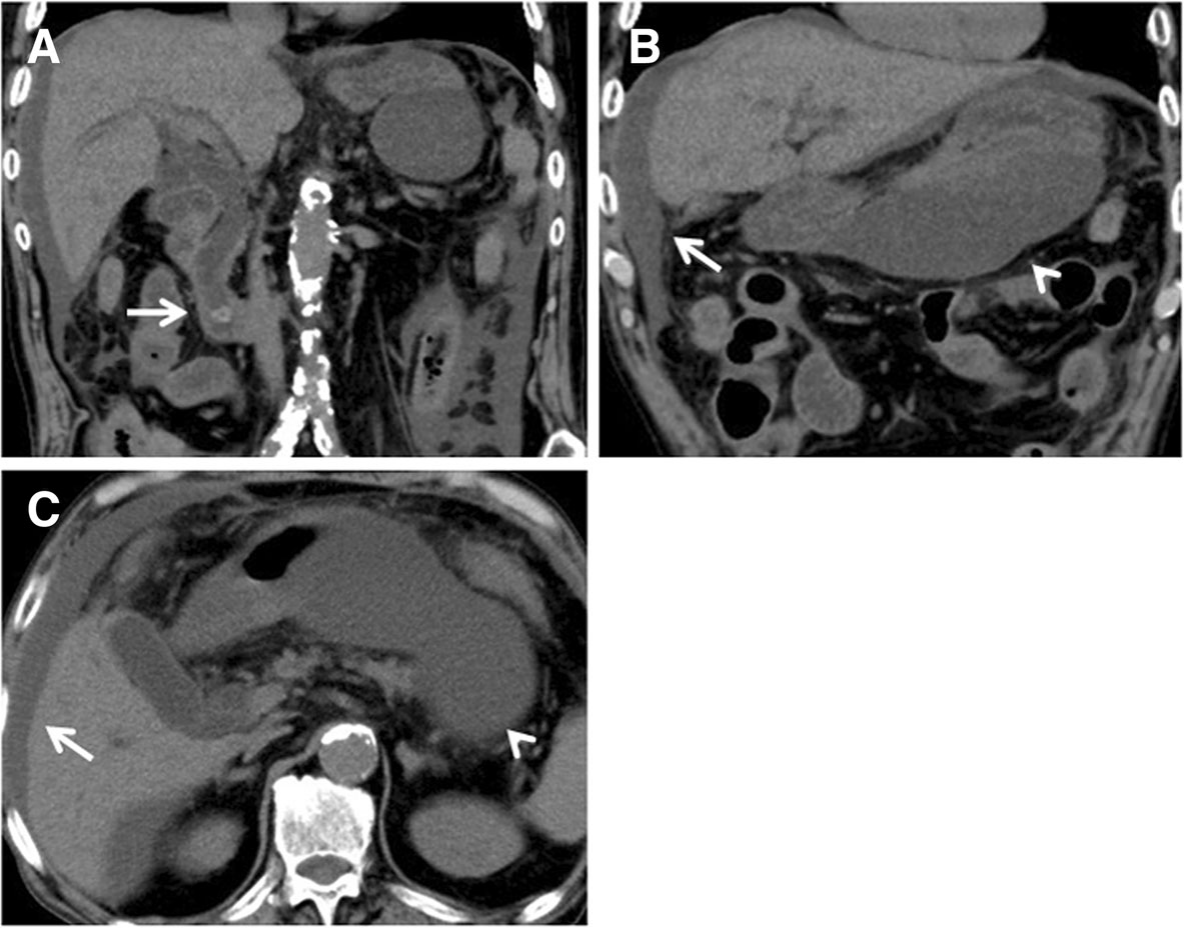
Computed tomography revealed common bile duct stones (a, *arrow*) and massive ascites in the omental bursa (b, c, *arrowhead*) and around the liver (b, c, *arrow*)
